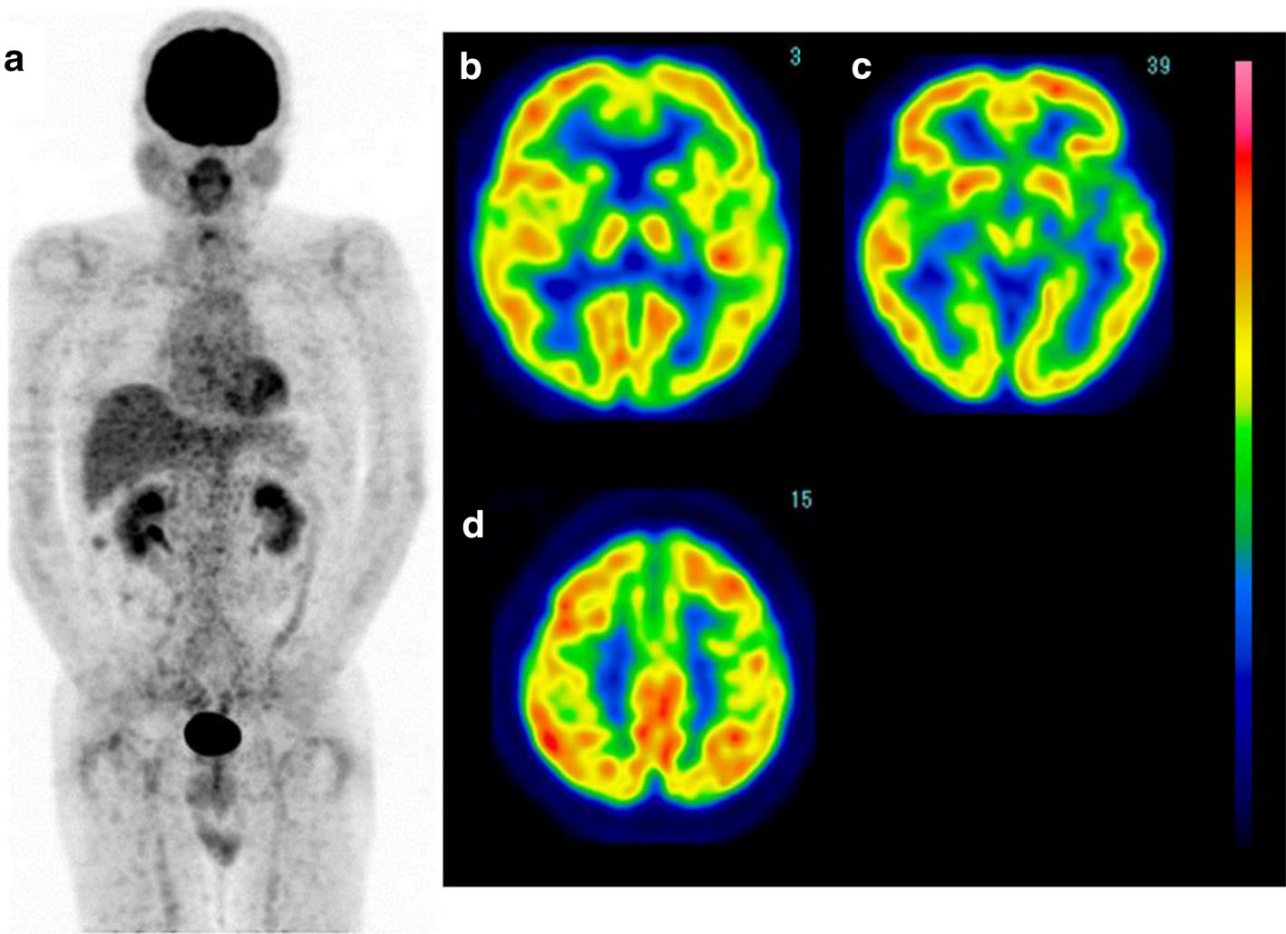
After chemotherapy, the abnormal FDG uptake in the liver and bone marrow disappeared (a) and the cerebral glucose metabolism improved (b–d)
